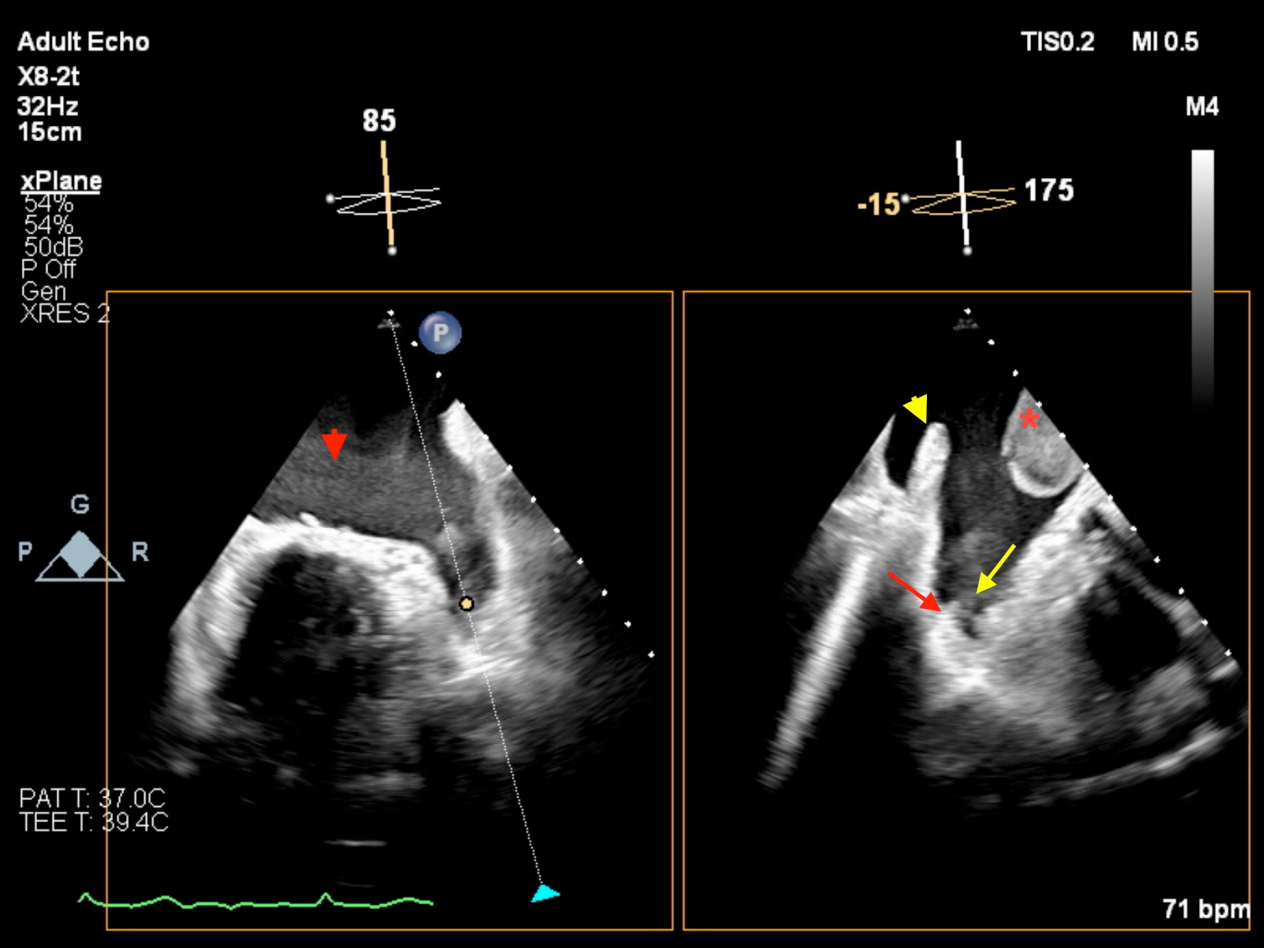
Biplane view in transesophageal echocardiography demonstrating left atrial thrombosis left atrial smoke, left atrial appendage sludge, pectinate muscle in the left atrial appendage, and Coumadin ridge. Red arrow: Pectinate muscle; Yellow arrow: Left atrial appendage sludge; Red arrowhead: Left atrial smoke; Yellow arrowhead: Coumadin ridge; Red star: Left atrial thrombosis.
